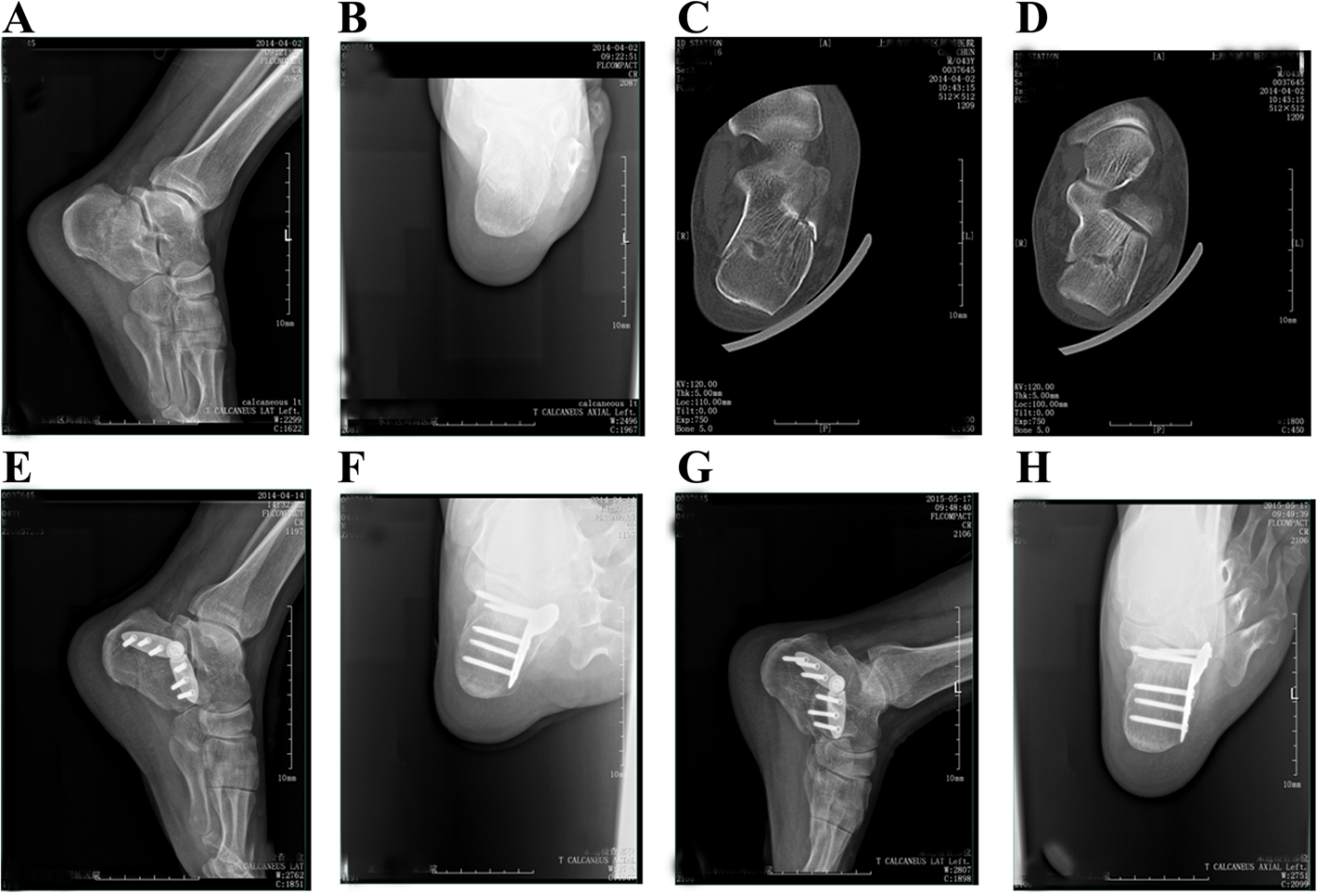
A 45-year-old male patient was admitted to our hospital because of pain and swelling on the left foot after falling from a height. Preoperative lateral (a) and axial (b) radiographs showing the left calcaneal fracture, with reduced calcaneal height (34.5 mm) and length (62.3 mm) but increased calcaneal width (39.4 mm). Preoperative horizontal (c) and coronal (d) computed tomography images showing a Sanders IIA fracture with a bulked lateral wall. Postoperative lateral (e) and axial (f) radiographs showing obvious corrections of the calcaneal height (38.6 mm), length (67.5 mm), and width (39.3 mm). Postoperative lateral (g) and axial (h) radiographs showing excellent fracture union, with a Maryland foot score of 88
